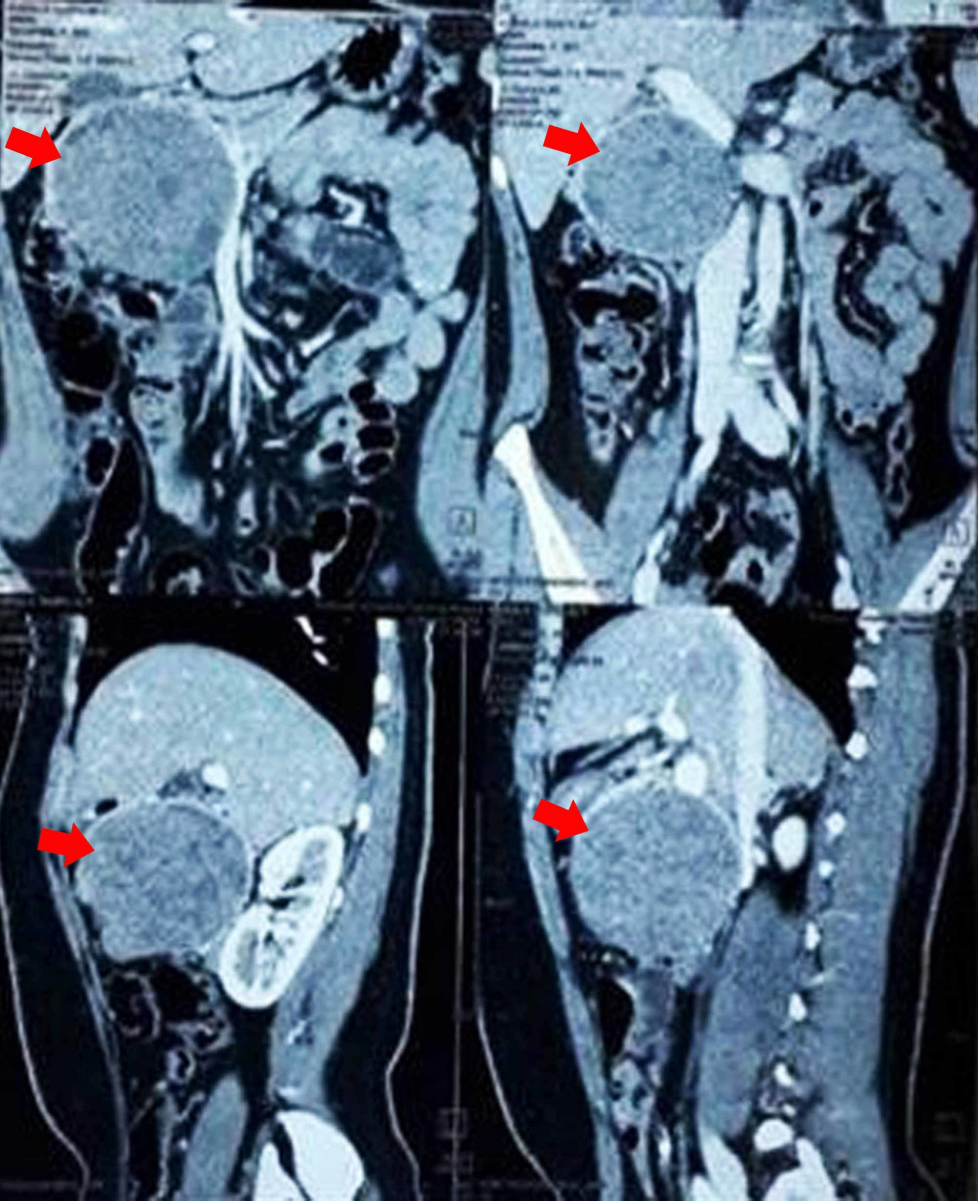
CECT showing mass in the pancreatic head (red arrows) CECT, contrast-enhanced computed tomography
